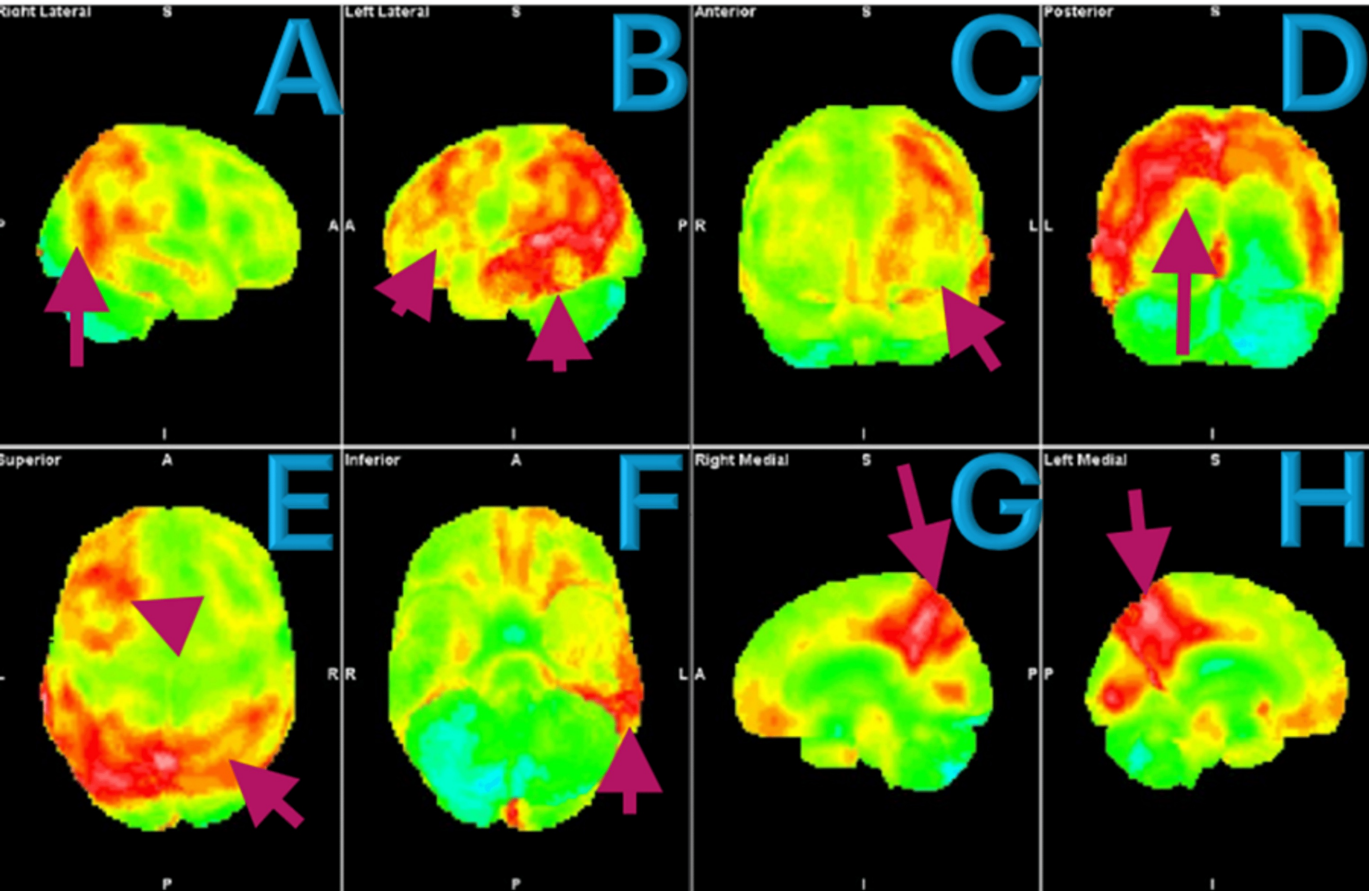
Amyloid PET-CT of the brain (A, B) Right and left lateral views of the brain, respectively. (C, D) Anterior and posterior views. (E, F) Superior and inferior views. (G, H) Right and left sagittal views. The arrows represent amyloid deposition that can be appreciated primarily in the occipital and temporal lobes. In B and E, there is some amyloid deposition localized to the frontal lobes.
